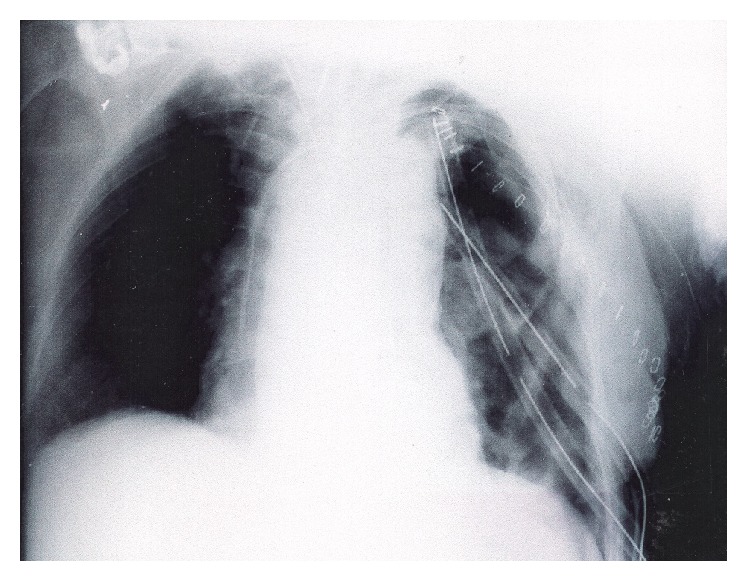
The postoperative chest X-ray showing reexpansion of the left lung.
